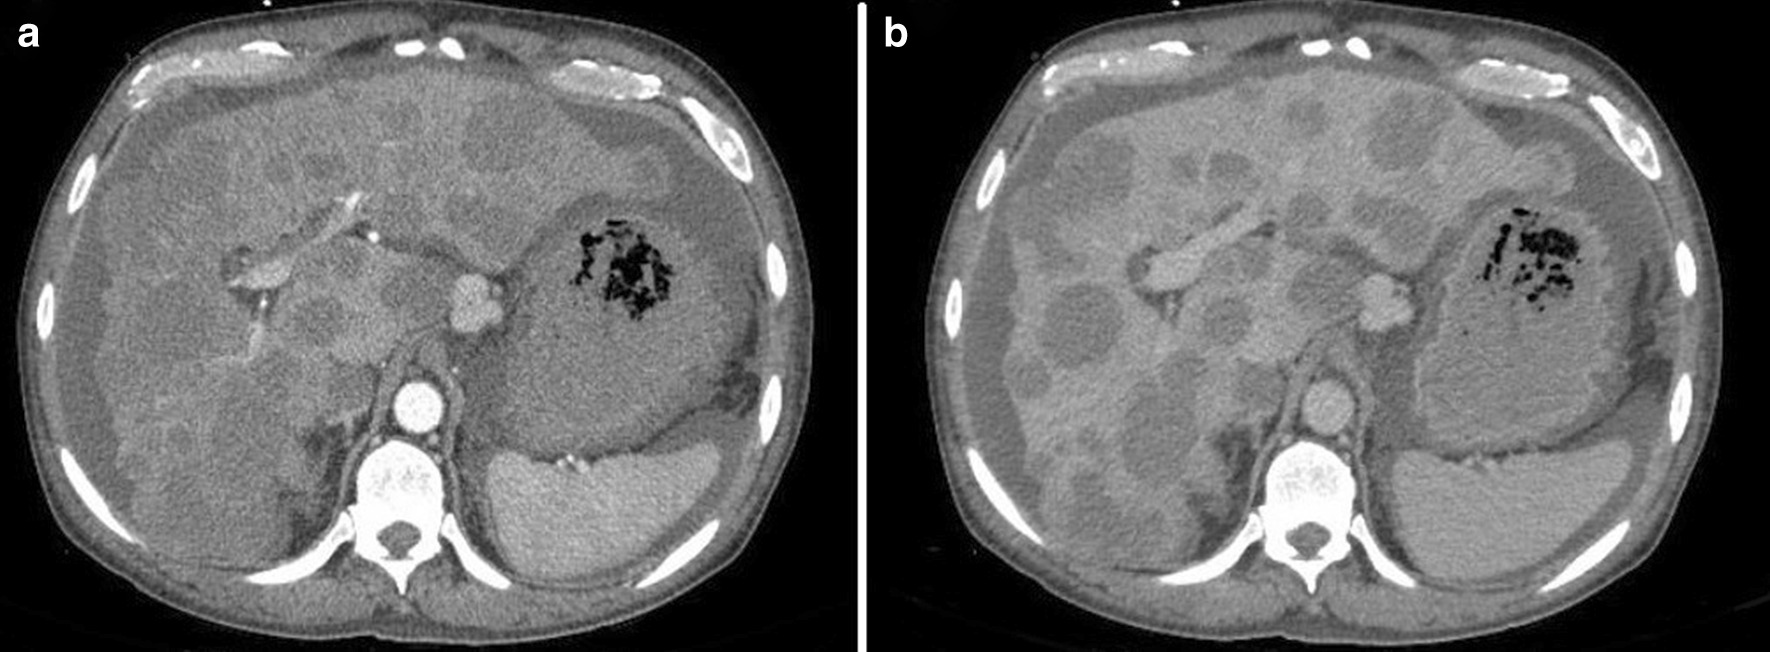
Cross sectional imaging CT scan abdomen demonstrates multiple hypodense hepatic lesions, a arterial phase. b Portal venous phase
